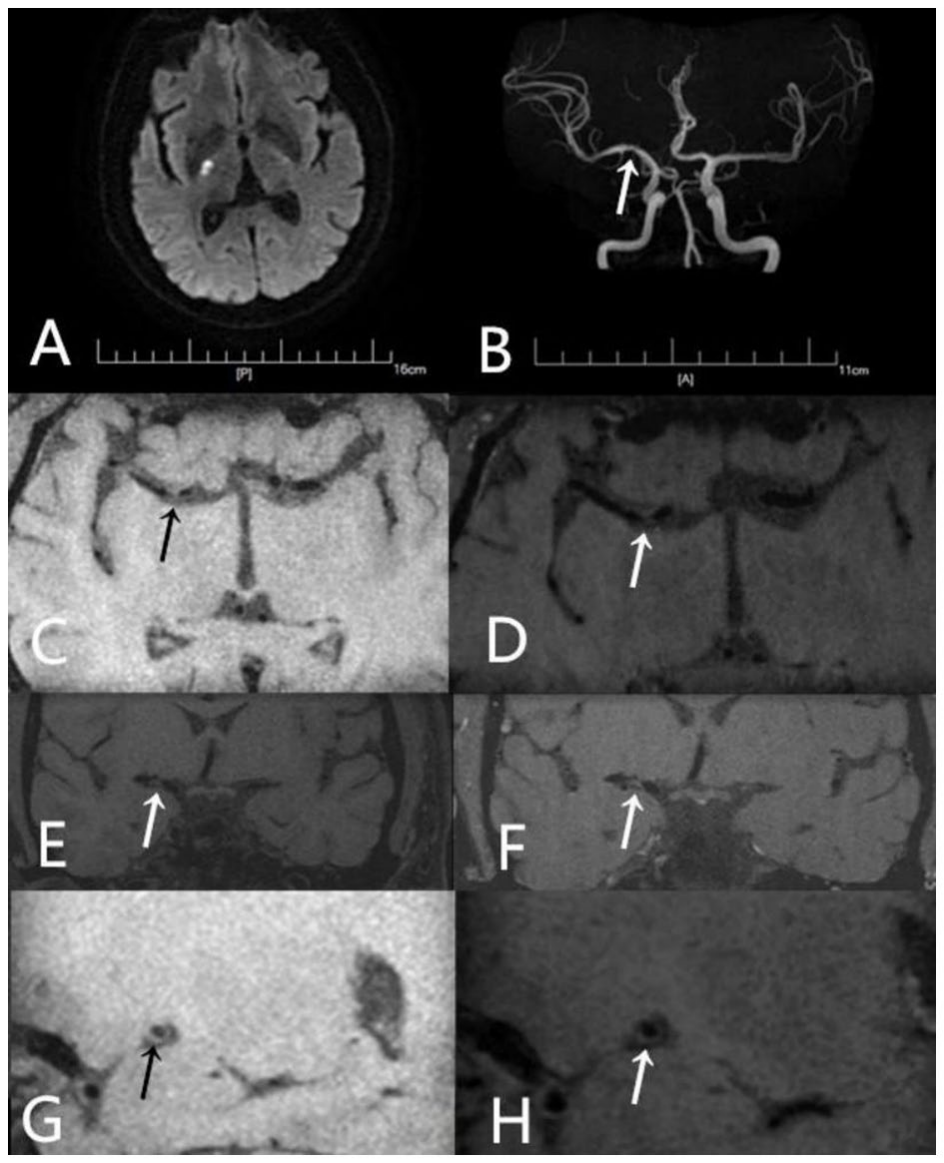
A 55-year-old male patient was admitted to the hospital due to left-sided hemiparesis for 5 days. Prior to the onset of symptoms, he had been taking Atorvastatin 20 mg once daily. (A) DWI shows a high signal intensity lesion in the right basal ganglia region. (B) TOF-MRA shows mild stenosis in the right middle cerebral artery (arrow). (C) Axial T1-weighted pre-contrast images shows the plaque (arrow). (D) Axial T1-weighted post-contrast images demonstrates eccentric wall thickening of the plaque (arrow) without enhancement. (E) Coronal T1-weighted pre-contrast image shows the plaque (arrow). (F) Coronal T1-weighted post-contrast image demonstrates eccentric wall thickening of the plaque (arrow) without enhancement. (G) Sagittal T1-weighted pre-contrast image shows the plaque (arrow). (H) Sagittal T1-weighted post-contrast image demonstrates eccentric wall thickening of the plaque (arrow) without enhancement.
